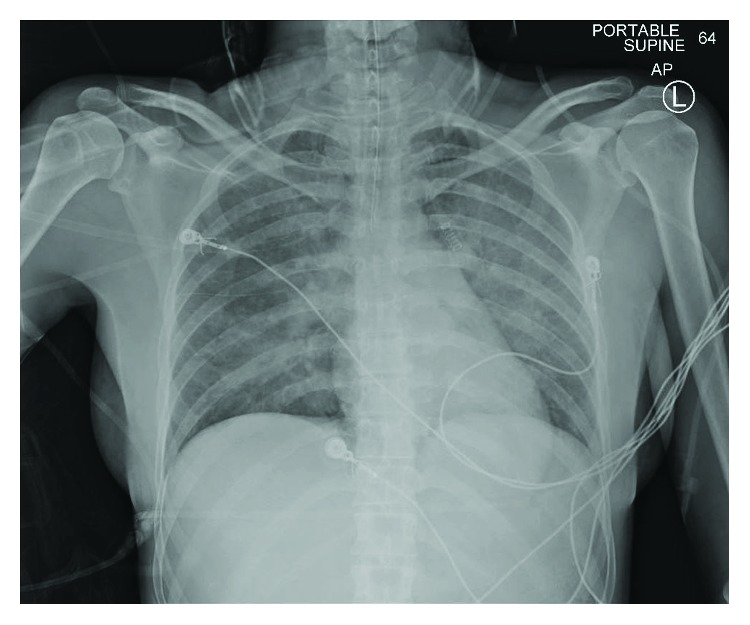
Chest radiograph demonstrating diffuse pulmonary edema upon admission to the emergency department.
